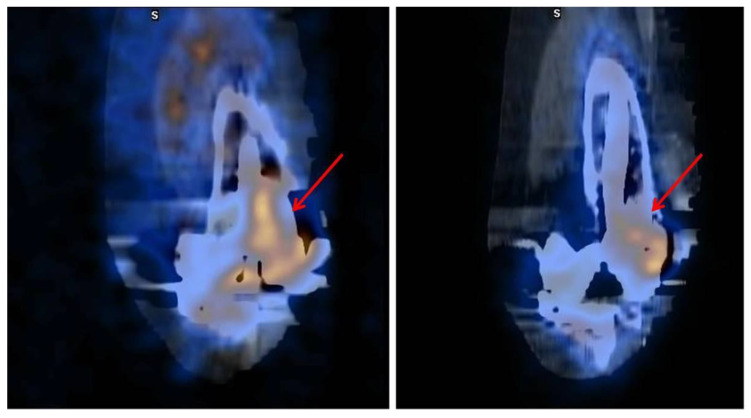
Prosthetic left knee infection detected by scintigraphy (^99m^Tc-HDP and ^67^Ga) and SPECT-CT. Combination of scintigraphy with ^99m^Tc-HDP and ^67^Ga with SPECT-CT in an 84-year-old male patient with prosthetic left knee infection and multiple comorbidities. The scan showed the focus of increased bone uptake in the external femoral condyle, with extensive associated gallium deposition, compatible with an infectious process (red arrows).
